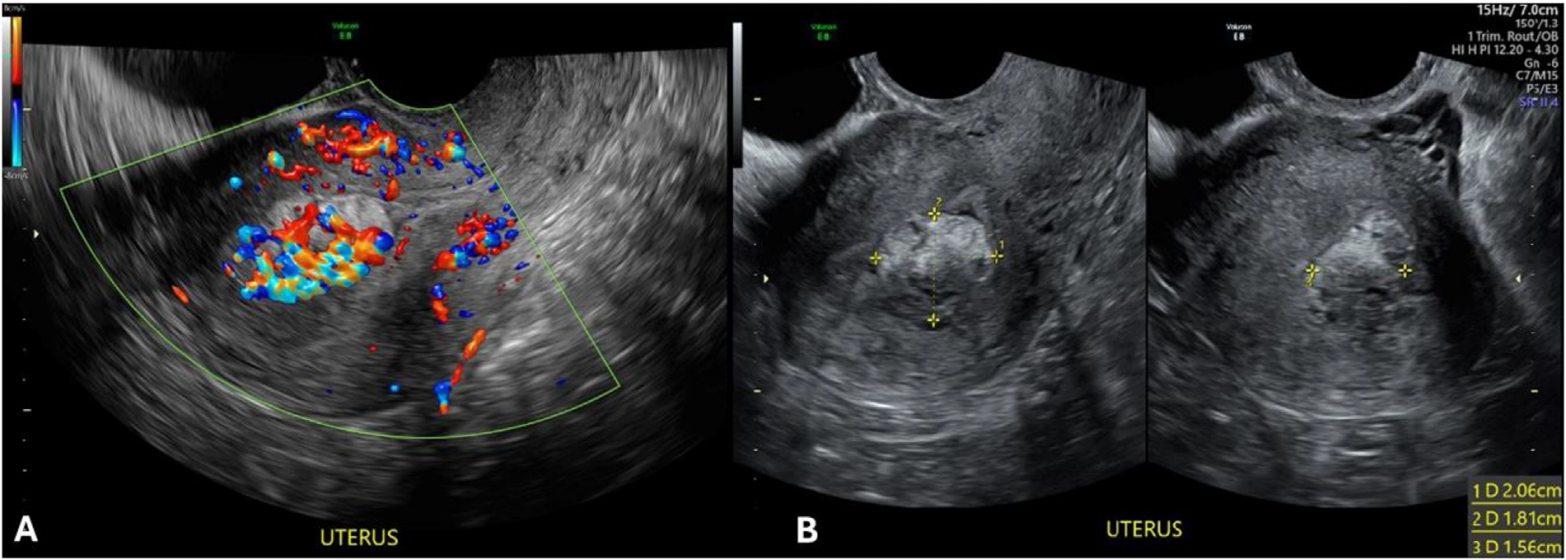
Transvaginal Ultrasound showing doppler findings (A) and spatial measurements of echogenic mass (B) Features suggestive of an increased vascular component that is indistinguishable whether it is located in the endmetrium or the myometrium.
